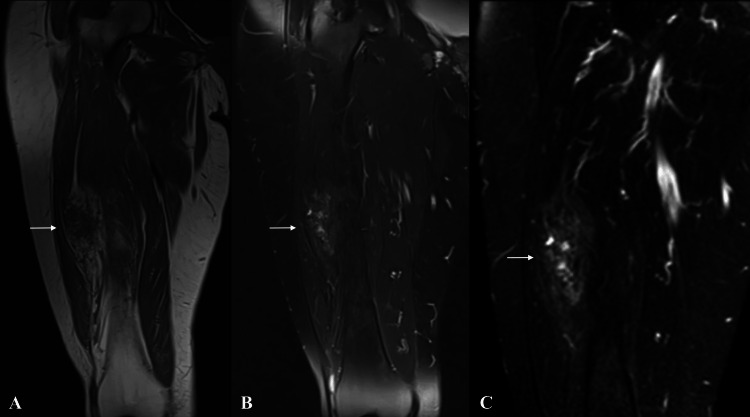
MRI of the patient (A) Coronal T1-, (B) T2-, and (C) gadolinium contrast T2-weighted images showing a relatively well-defined mass in the vastus intermedius (arrow). The lesion is streaky with lines of fatty and fibrous tissue MRI: magnetic resonance imaging
